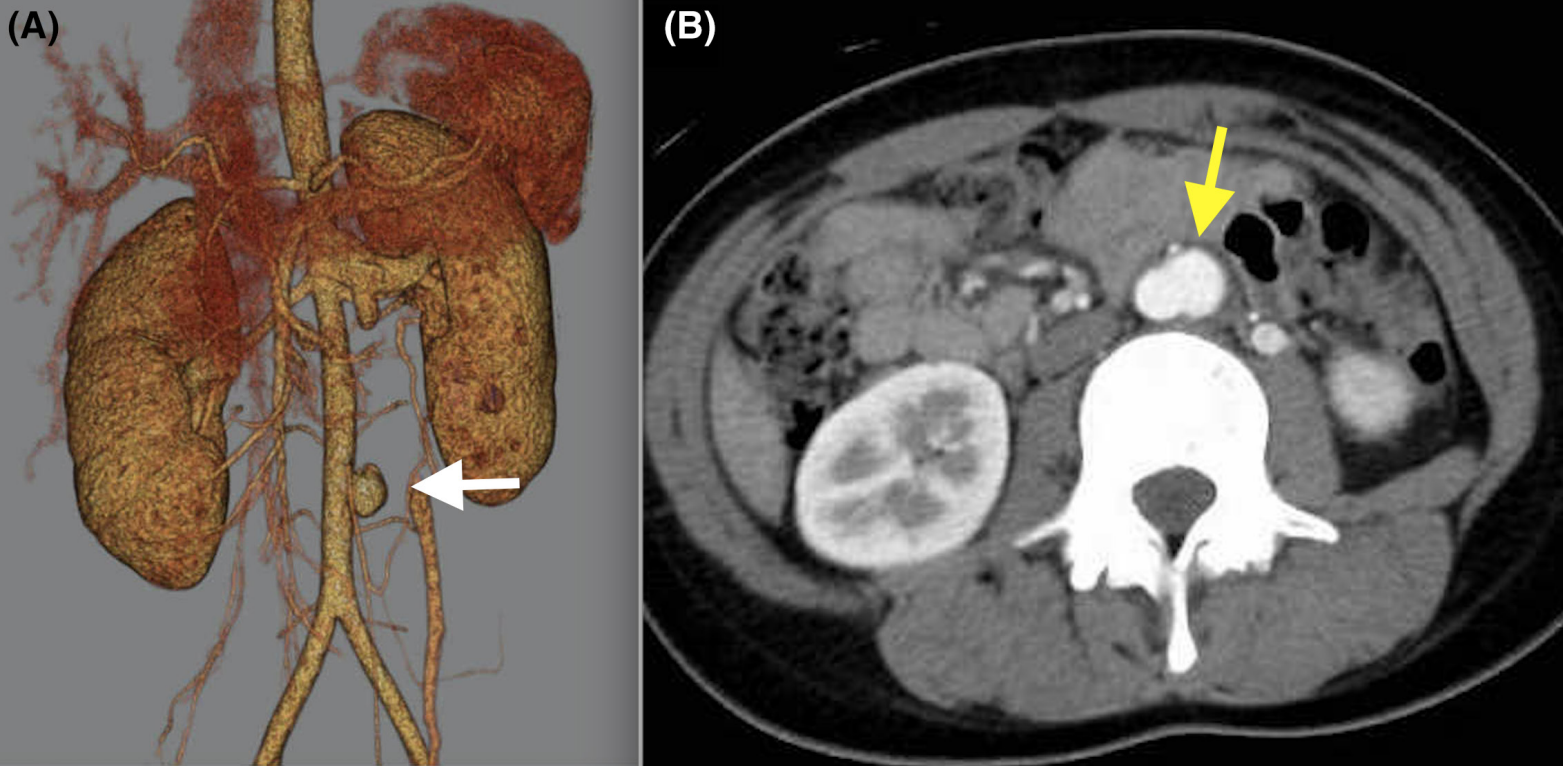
The 3D computed tomography showed an infrarenal abdominal aortic aneurysm of 19 × 18 mm (white arrow). (A), Computed tomography showed a saccular abdominal aortic aneurysm on the left side of the infrarenal abdominal aorta (yellow arrow) (B).
